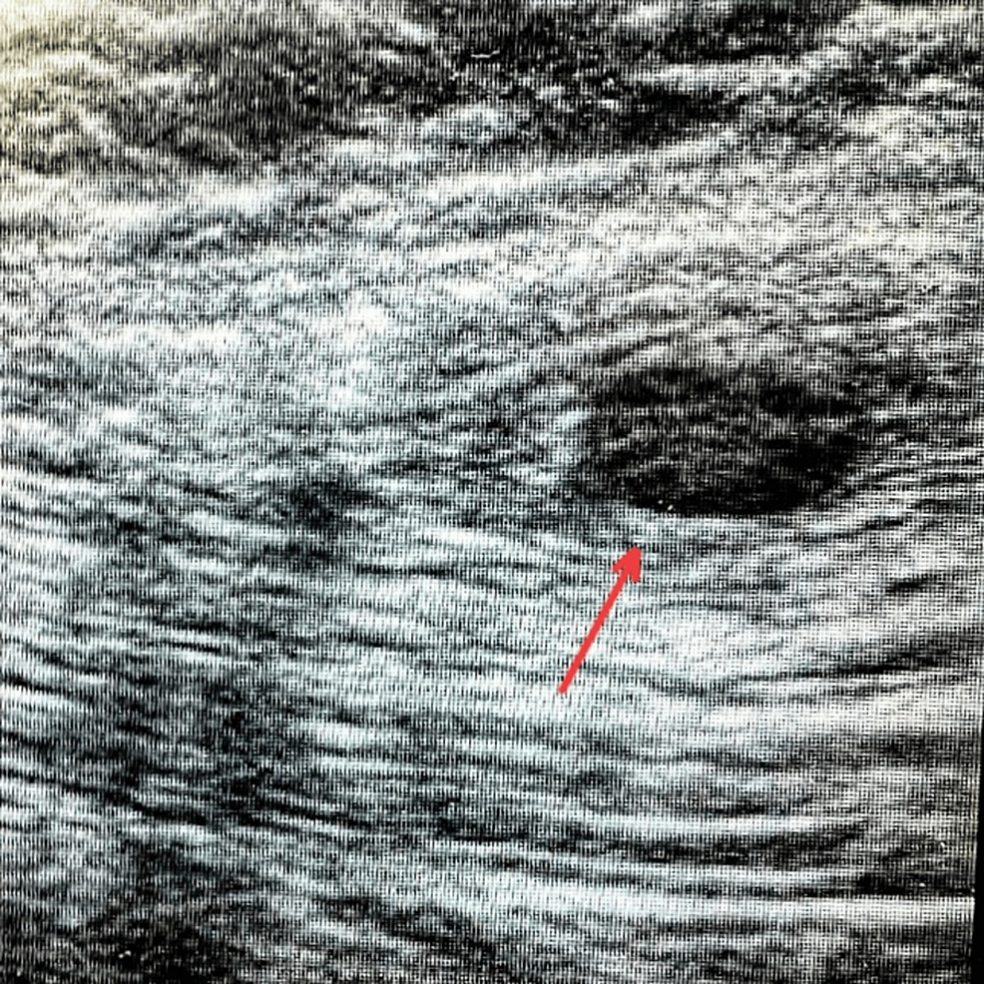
Intraoperative ultrasonography The red arrow shows a hypoechoic lesion - myocysticercosis.
